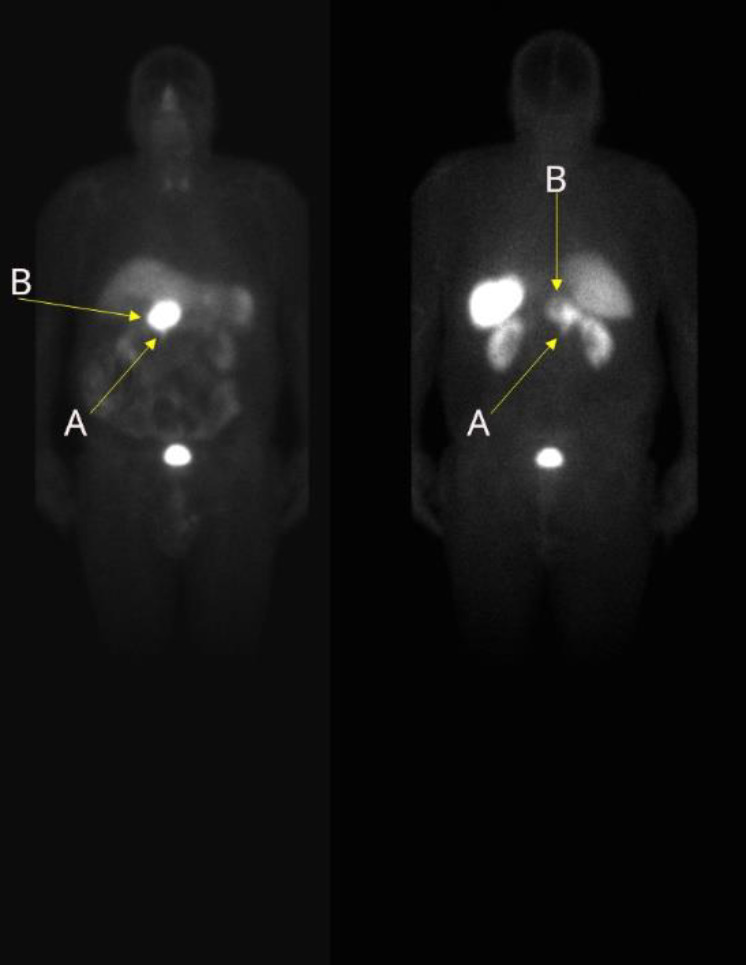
Planar ^99m^Tc-Tektrotyd® scintigraphy of Case 2, showing two lesions with high SSTR expression: (A) duodenal primary tumor, (B) peripancreatic lymph node metastasis
